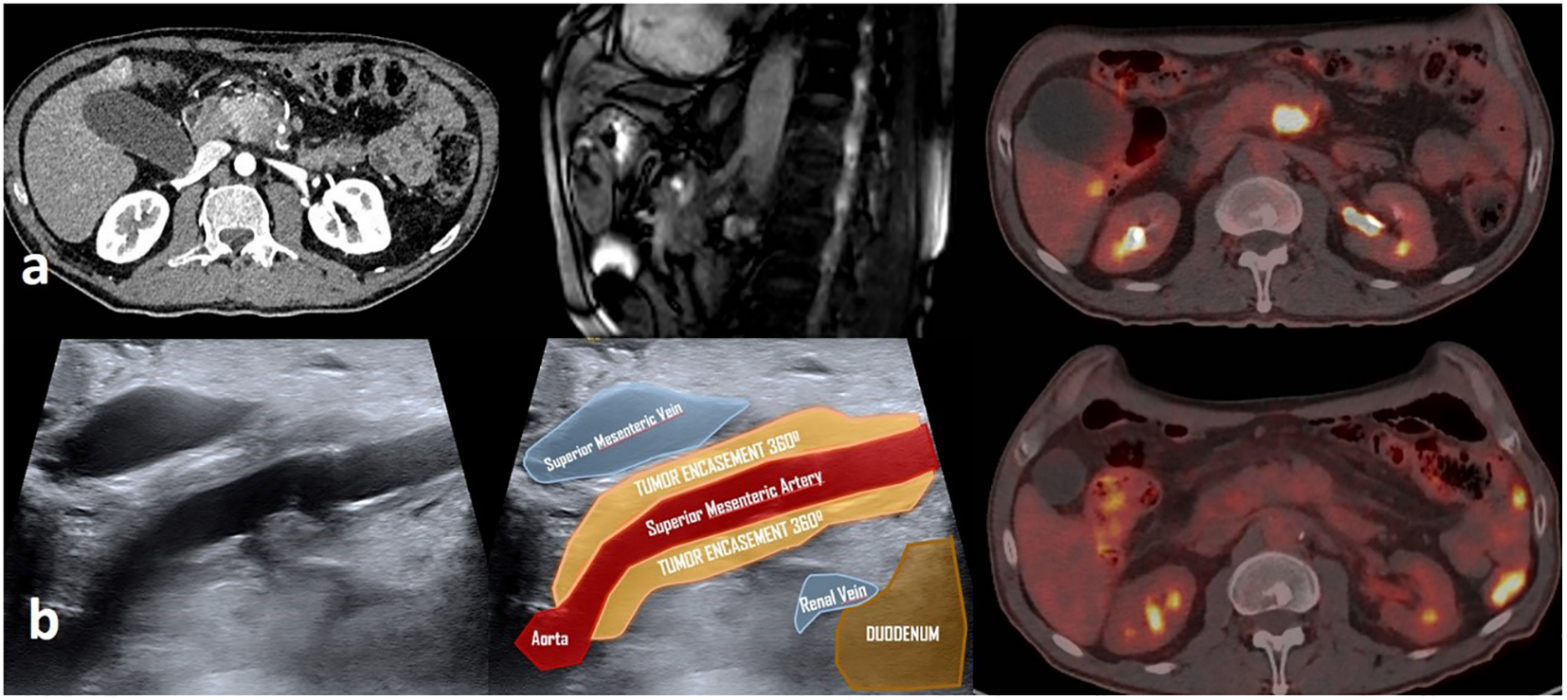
Imaging studies evolution in a patient with unresectable pancreatic cancer treated with induction chemotherapy FOLFIRINOX, neoadjuvant chemo-radiation with proton therapy and IOeRT boost consolidation. (A) Initial diagnostic studies (angioCT, MRI, and PET-CT) showing the vascular involvement and unresectable nature of the disease. (B) Post-treatment imaging studies showing a complete metabolic remission and a 360° encasement of the superior mesenteric artery.
